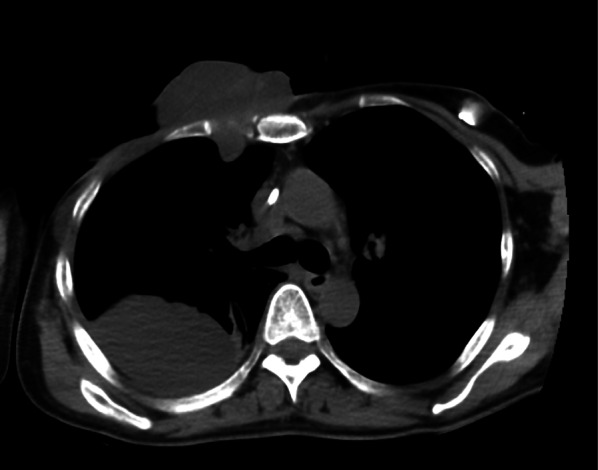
Computed tomography revealed regrowth of tumor in the right chest, right lung and right pleural effusion
